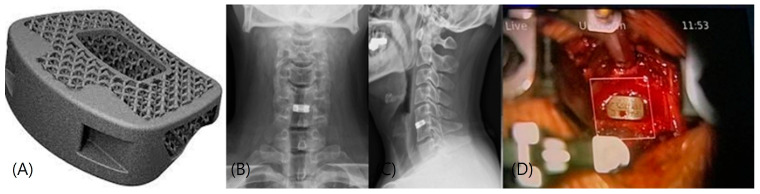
Surgical procedure and implant. (A) Stand-alone 3D-printed porous titanium cage. (B) Anteroposterior radiograph showing cage position. (C) Lateral radiograph demonstrating proper cervical alignment. (D) Intraoperative photograph showing the cage filled with i-Factor™ inserted in the disc space. All images are original captures from this study cohort. Representative case: a 53-year-old female with right-sided C5–6 disc herniation and C6 radiculopathy refractory to conservative treatment who underwent single-level C5–6 ACDF using a stand-alone 3D-printed porous titanium cage filled with i-Factor™.
